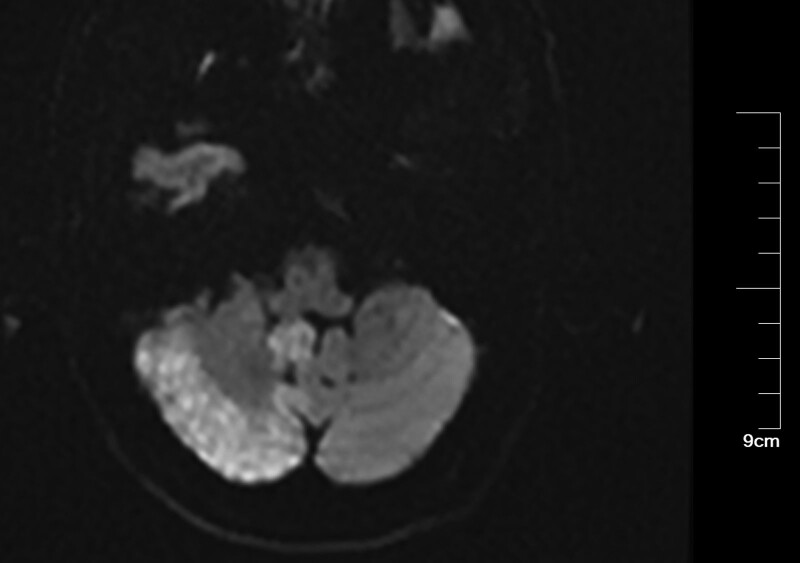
Imaging after cerebral angiography. DWI revealed a high-intensity lesion in the right cerebellum. DWI = diffusion-weighted imaging.
